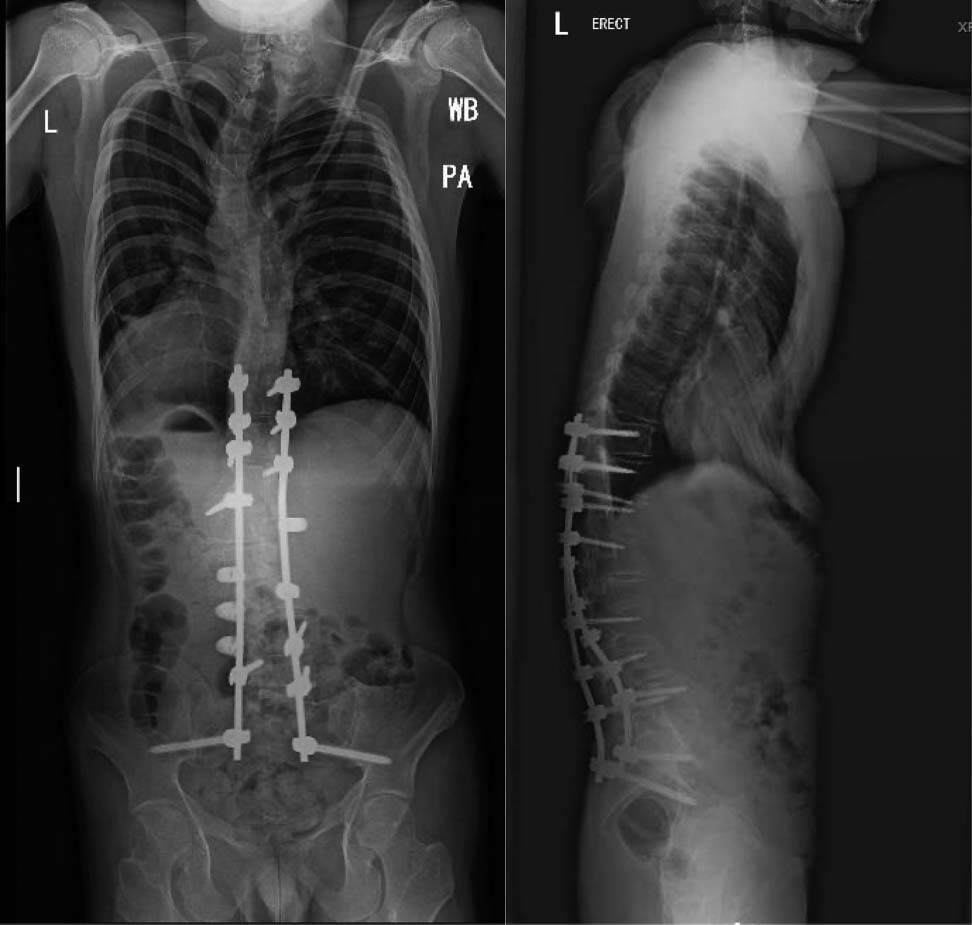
Posteroanterior and lateral plain radiographs demonstrating stable proximal junctional kyphosis and construct at 3-year follow-up.
